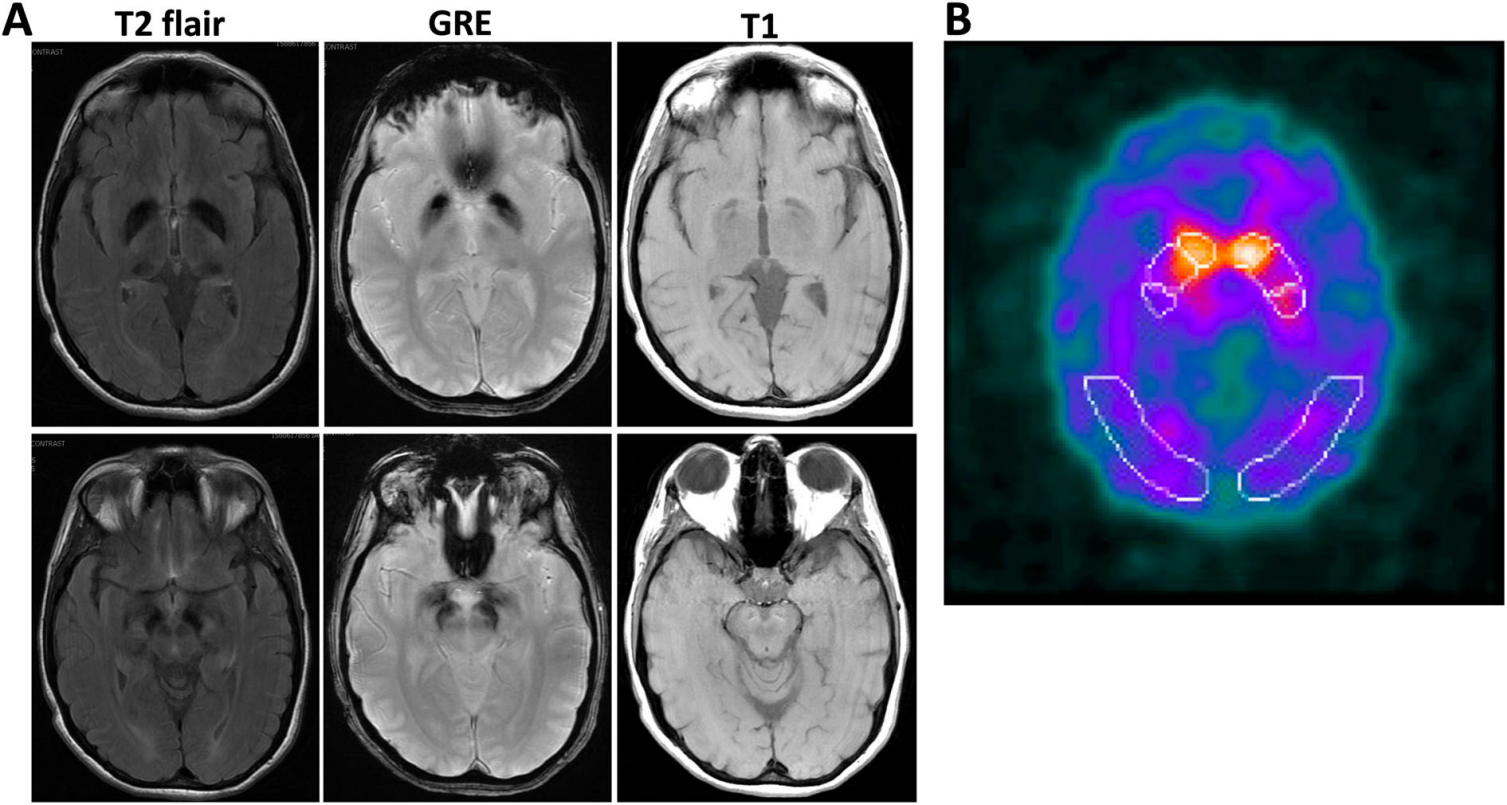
(A) 123 I‐Ioflupane single‐photon emission computed tomography (SPECT) showing bilateral decreased radiotracer uptake slightly more in the right putamen. (B) Axial T2‐weighted and multiecho gradient recalled echo (GRE) T_2_*‐weighted magnetic resonance imaging (MRI) showing bilateral hypointense signal in the substantia nigra and globus pallidus showing bilateral hypointense signal mainly in the substantia nigra but also in the globus pallidus, indicating iron deposits. Axial T1‐weighted MRI showing the substantia nigra as a thin hypointense linear region, with a partial hyperintense halo surrounding it.
